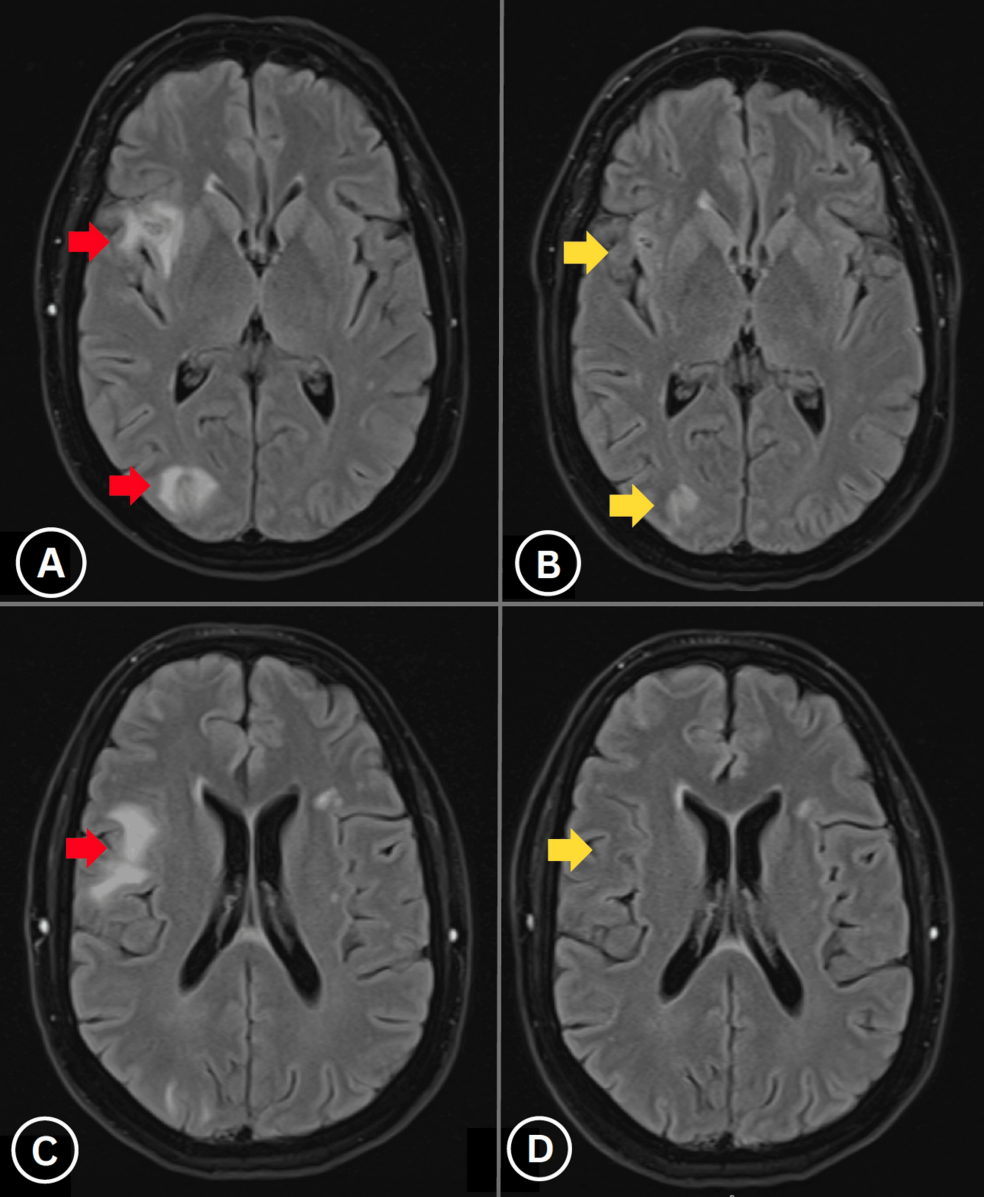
Post-corticosteroid treatment response. (A, C) Pre-treatment MRI at two weeks showing inflammatory lesion progression. (B, D) Post-corticosteroid MRI at five weeks demonstrates significant lesional regression (red arrows), confirming steroid-responsive inflammatory process.
